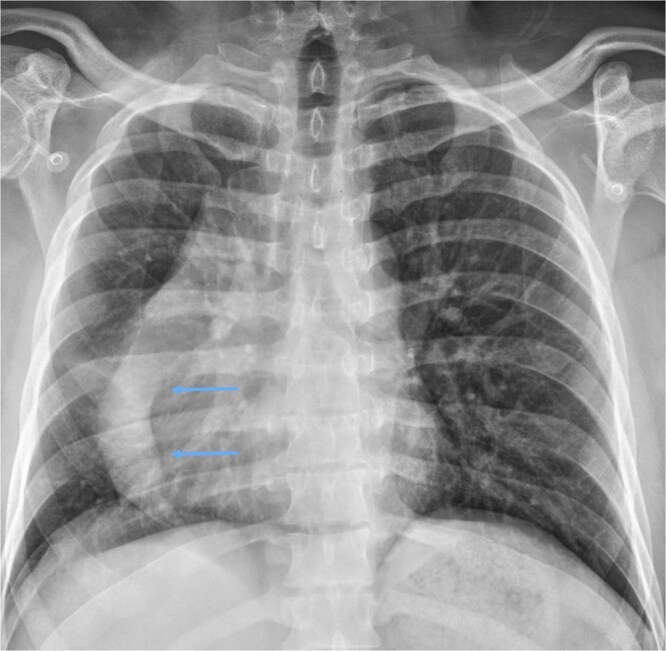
The chest X-ray shows a scimitar-shaped tubular structure in the right lung (arrows) and cardiac dextroposition.
